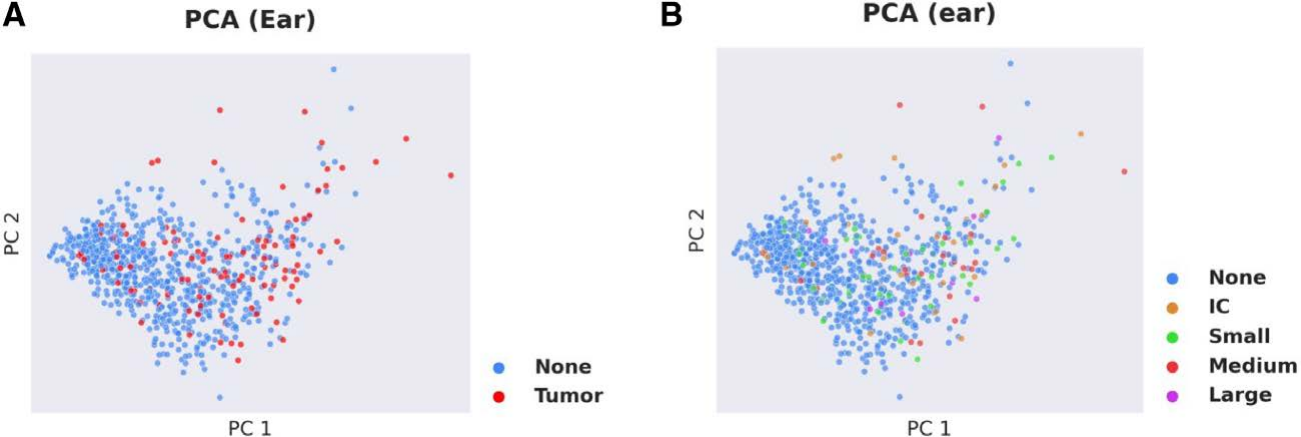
Deep-learning-based analysis of clinical and audiometric data in patients with and without vestibular schwannoma (VS) as confirmed by MRI.
